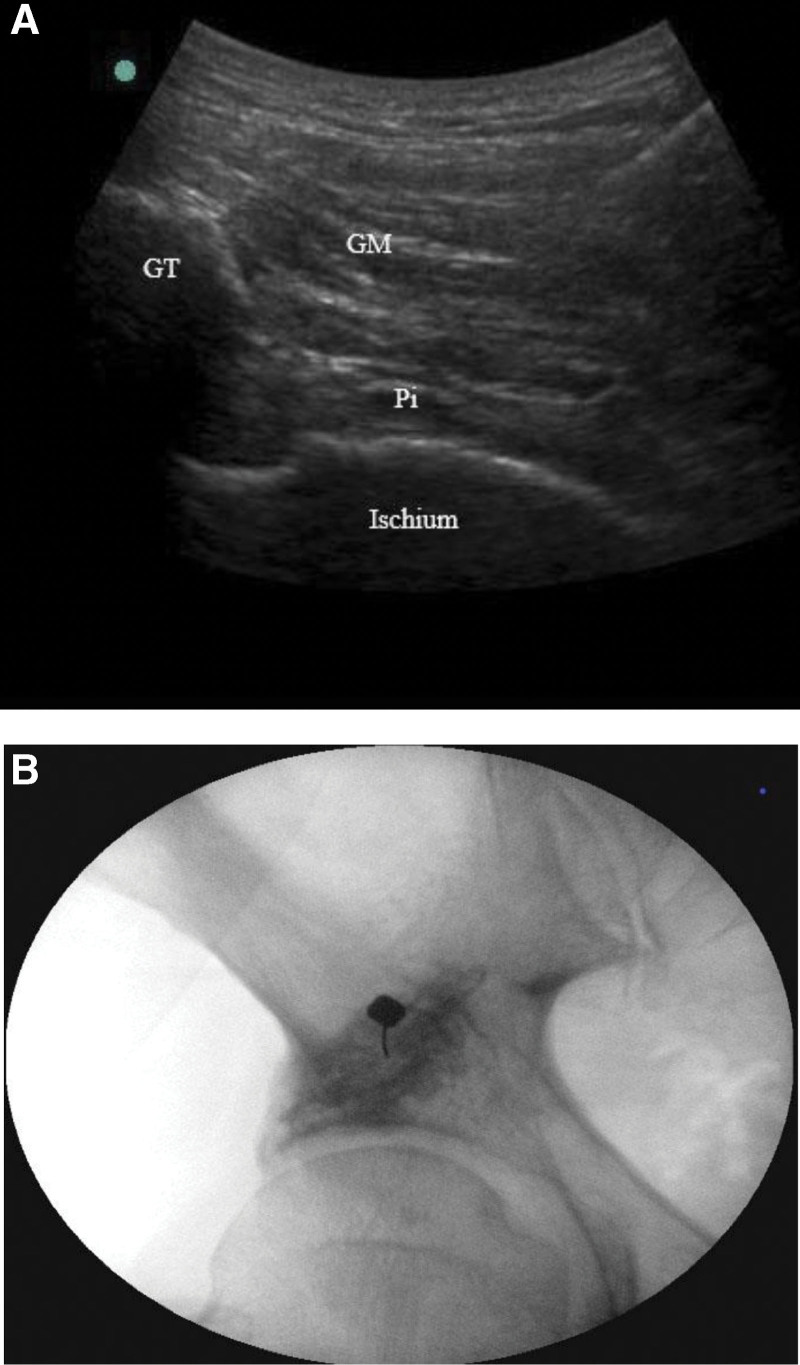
(A) Ultrasound image of the left buttock during targeted piriformis injection. (B) Fluoroscopic image of the piriformis muscle after contrast injection. GM = gluteus maximus, GT = greater tuberosity, Pi = piriformis.
